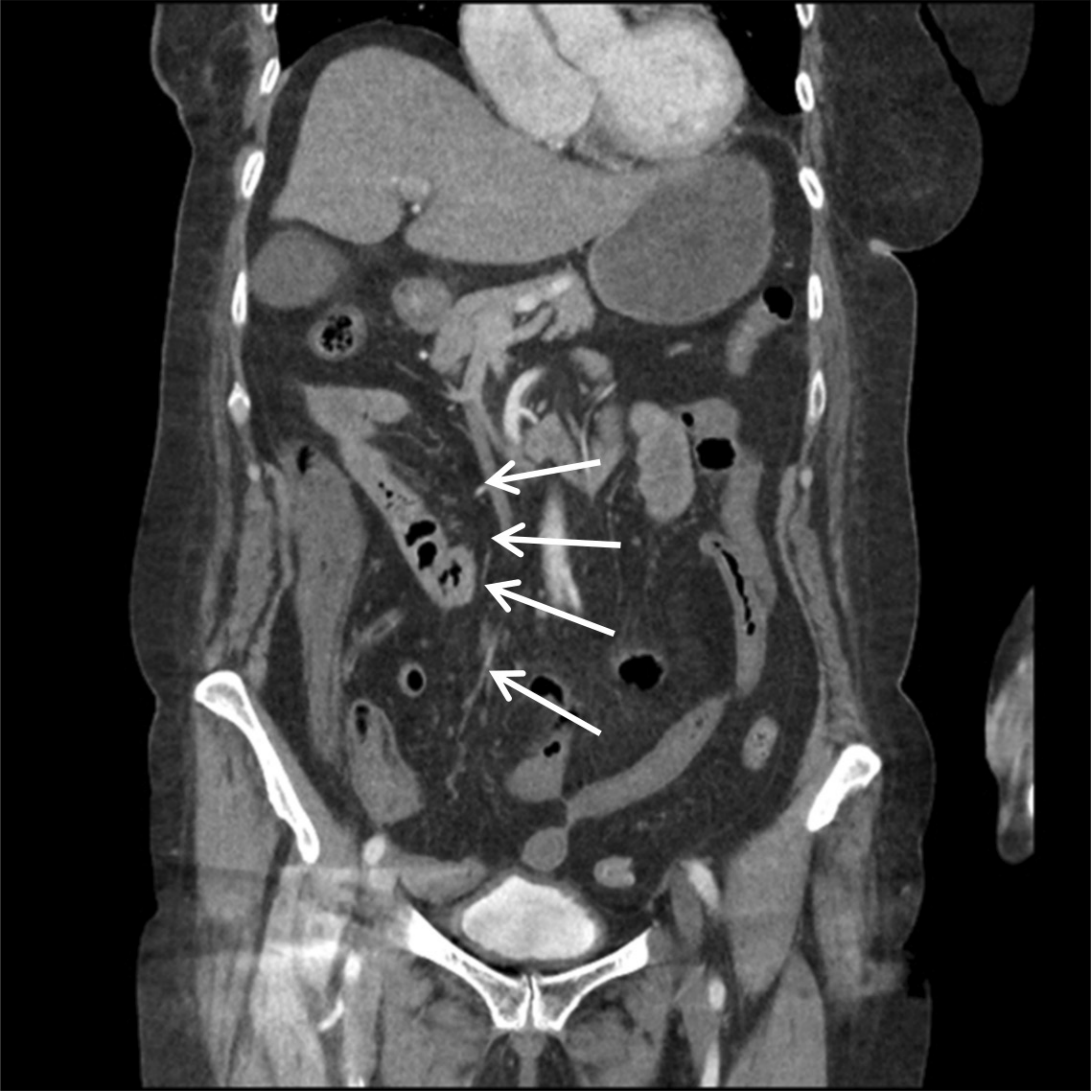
CT angiogram of the abdomen: Thrombus in superior mesenteric artery. Caecum and small bowel loops with apparent poor enhancement which may represent bowel ischaemia. 26/01/2021.
